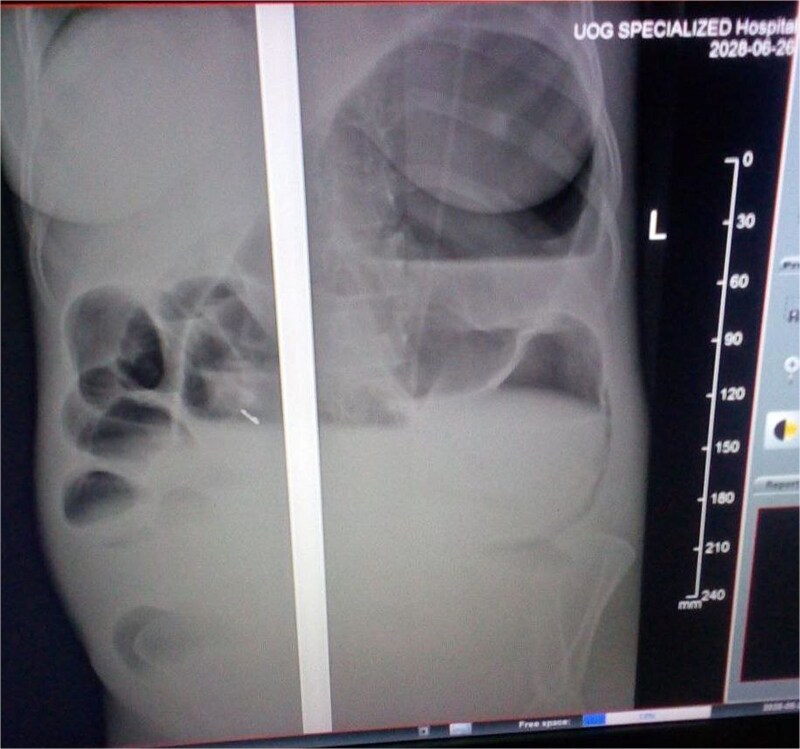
Abdominal X-ray showing the dilated colon and multiple air-fluid levels.
